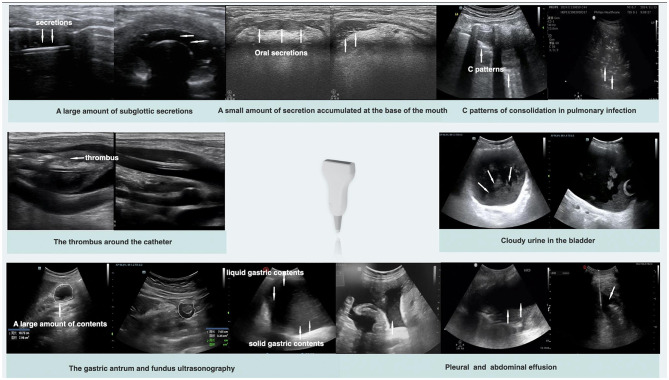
Typical ultrasound images of the five lines.
